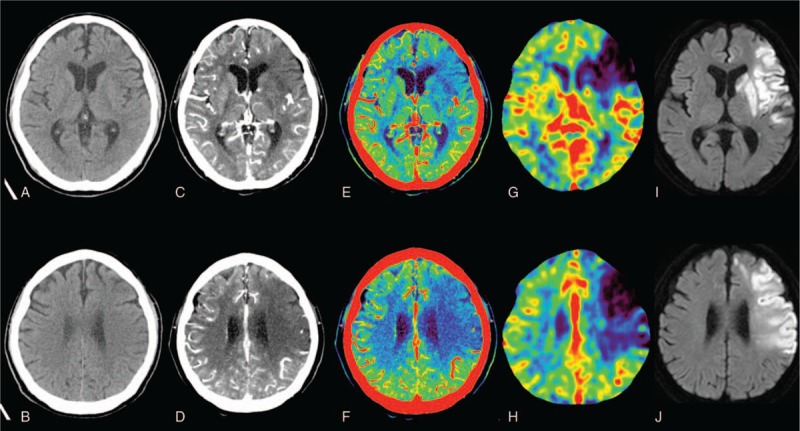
Onset NCCT (A, B), tMIP-gray (C, D), tMIP-color (E, F), CTP-CBV (G, H), and follow-up DWI (I, J) in representative patients with acute cerebral infarction caused by left ICA occlusion. This NCCT image is almost normal. However, the tMIP-gray and tMIP-color images clearly show low attenuation and avascular area in the left frontal-parietal lobe and basal ganglia, which correspond to low CTP-CBV areas. Follow-up DWI showed low-intensity area in the same location in which the onset NCCT shows EIC. CBV = cerebral blood volume, CTP = CT perfusion, DWI = diffusion weighted image, EIC = early ischemic change, ICA = internal carotid artery, NCCT = noncontrast computed tomography, tMIP = time maximum intensity projection CT technique.
